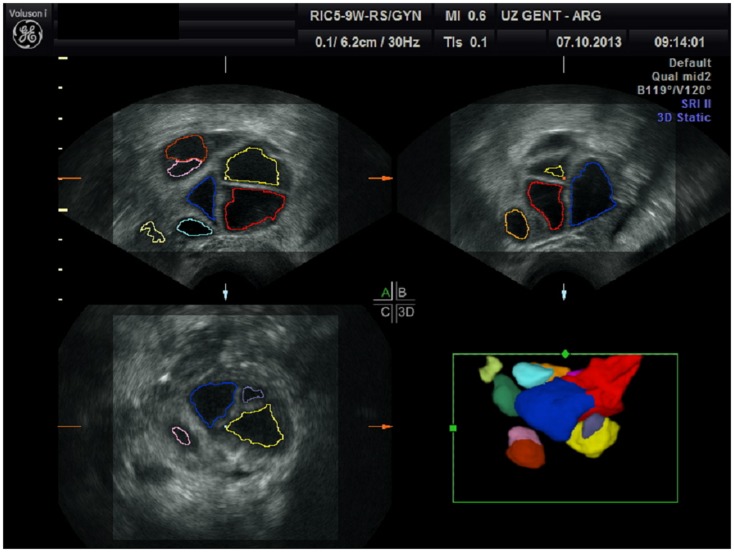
Follicular measurement using sonography-based automated volume count (SonoAVC).
